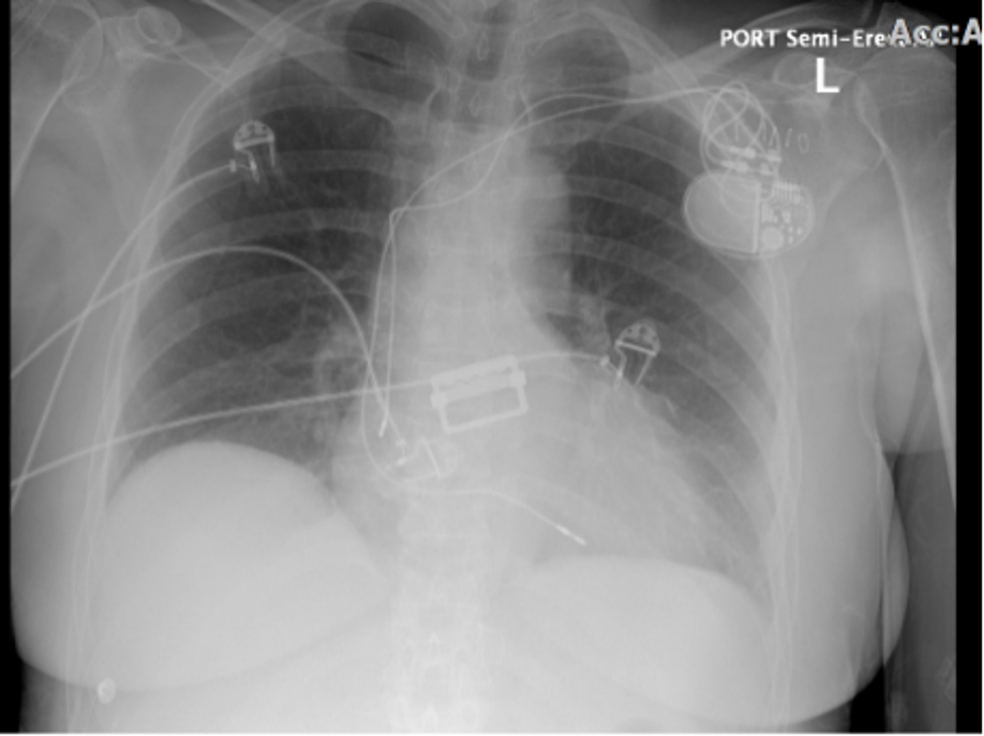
Chest X-ray showing proper placement of dual-chamber leads after pacemaker implantation
